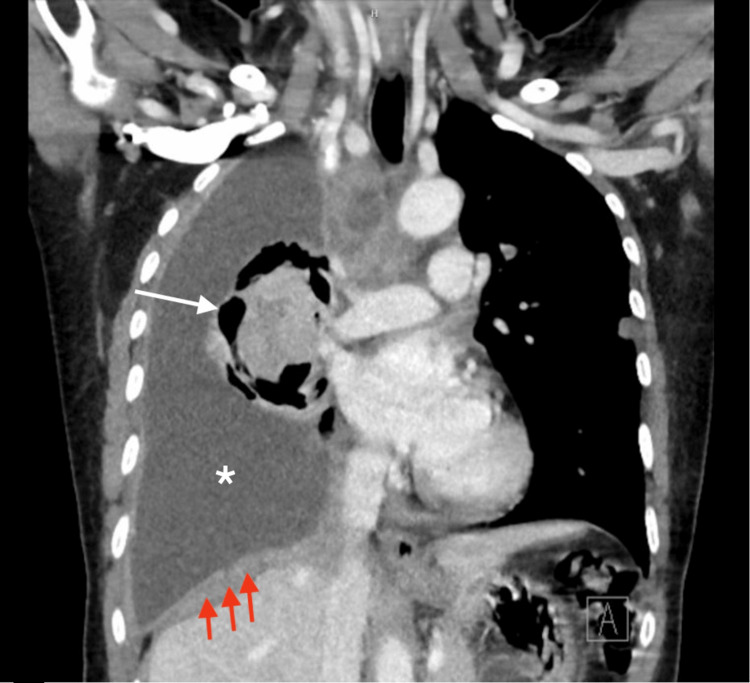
CT chest with contrast coronal view: irregular pleural thickening (red arrows), pleural effusion (white asterisk), and cavitary lesion (white arrow)
